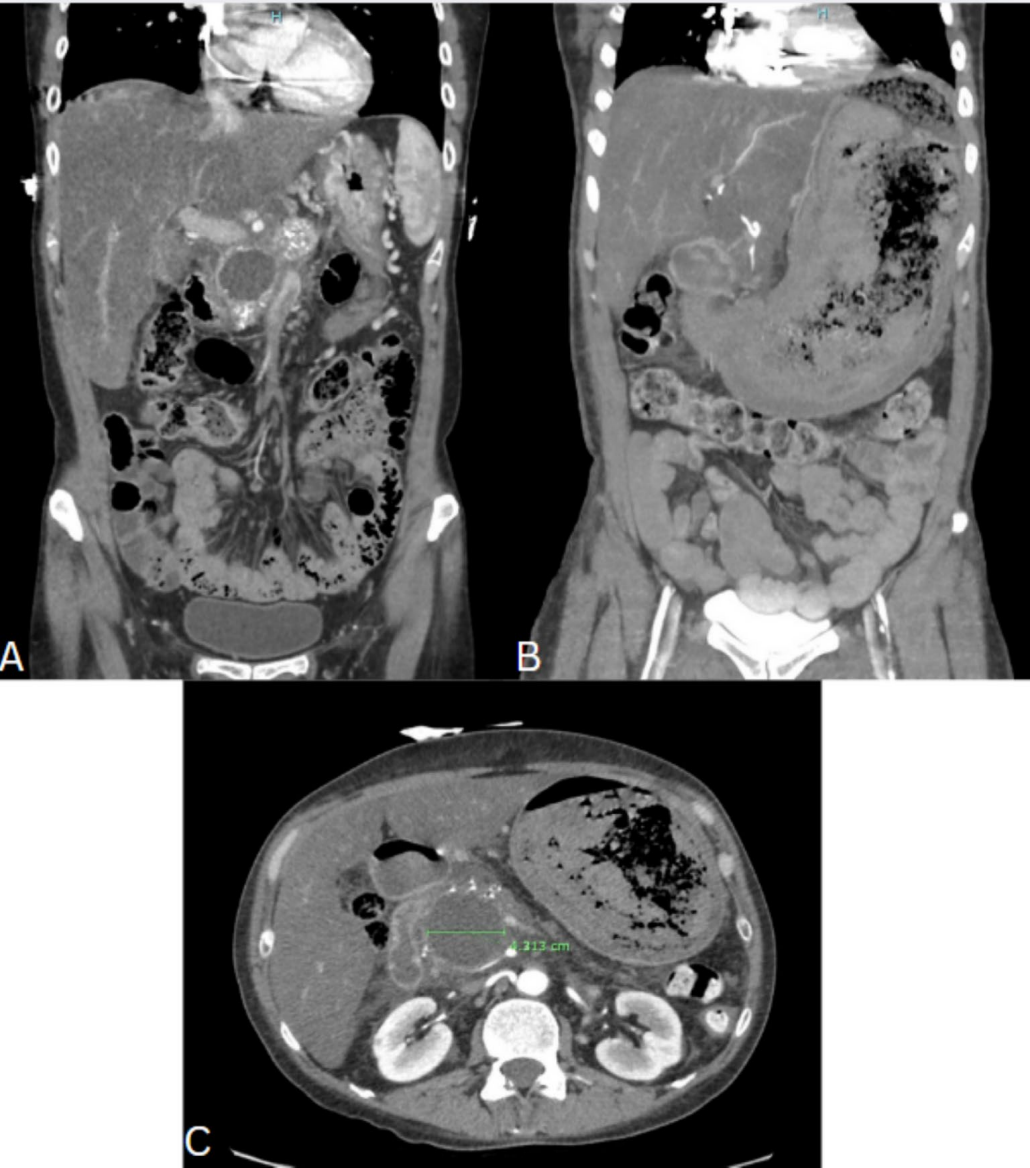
Initial CTA (coronal view) on admission for acute pancreatitis, demonstrating pseudocyst (A), followed by large amount of debris noted in the stomach on repeat CTA (coronal view) when transferred to the ICU on day 8 (B), and finally a 4.313 cm measured pseudocyst in the pancreatic head (C).
